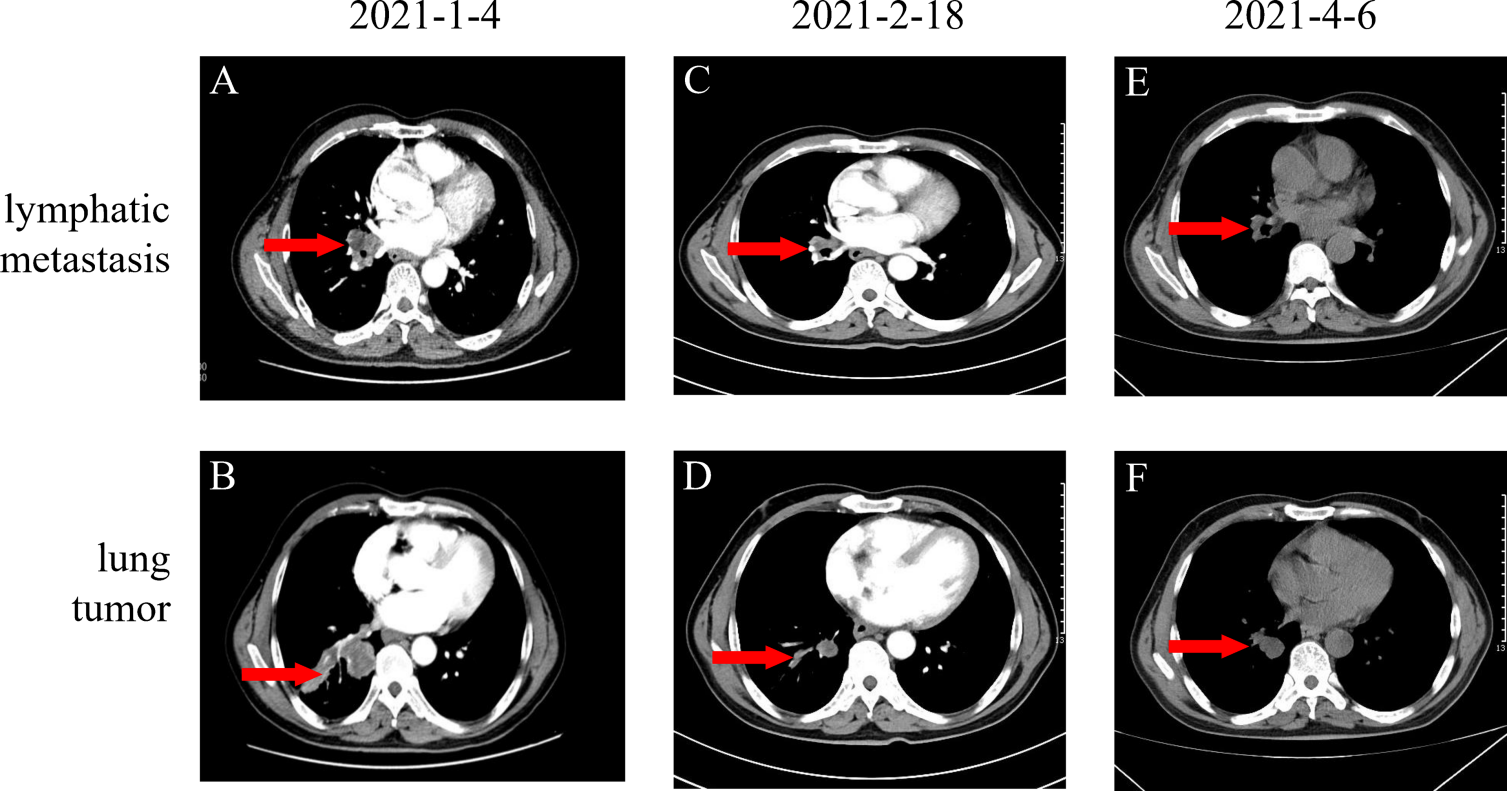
(A, B) Baseline images of CT before neoadjuvant therapy; (C, D) Original lung mass and lymphatic metastasis partial response (PR) after two cycles of AP (Albumin paclitaxel 400mg d1 and cis-platinum 60mg d1-d2) and Camrelizumab 200mg d1 (E, F) Original lung mass and lymphatic metastasis stable disease (SD) after four cycles of neoadjuvant therapy totally.
